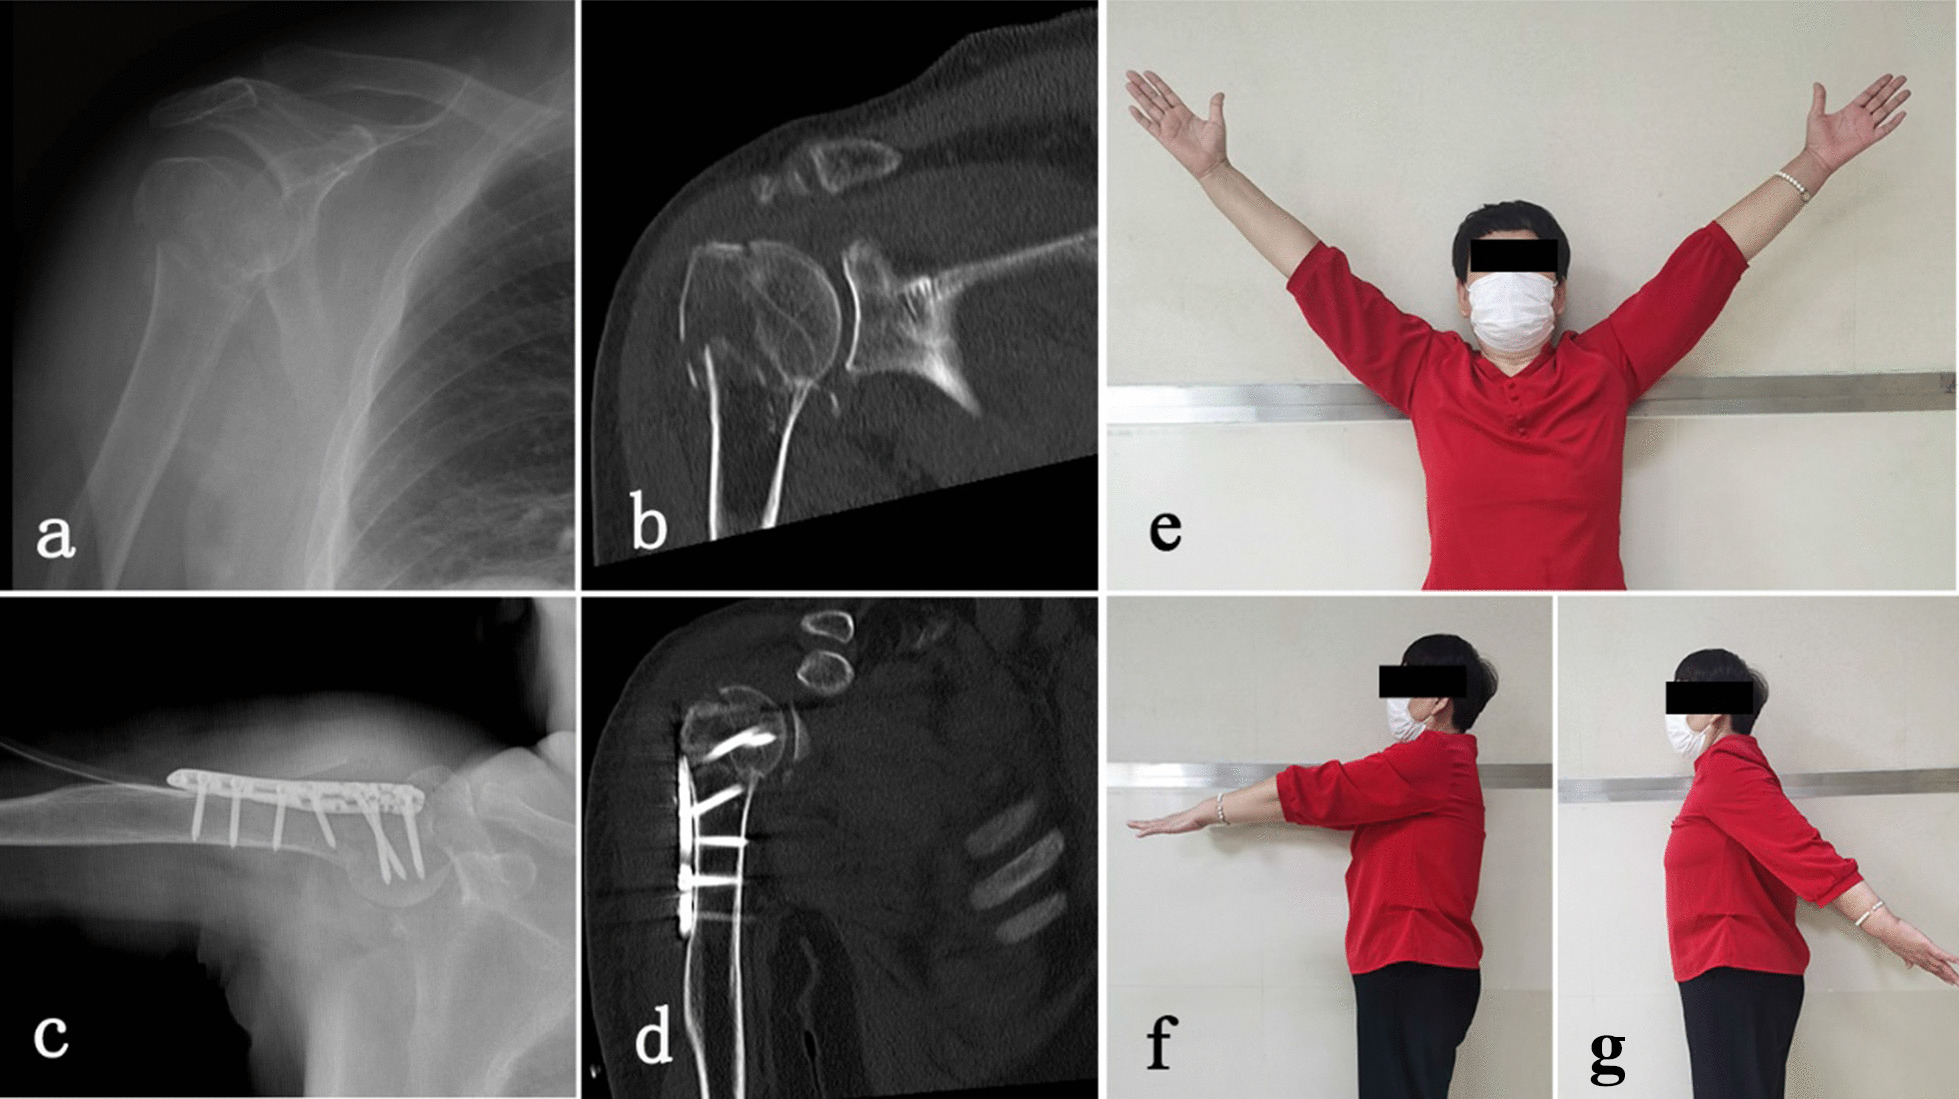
Patients with PHFs were treated using the locking plate approach. a Preoperative X-ray of the shoulder joint; b preoperative CT; c Postoperative X-ray; d Postoperative CT scan of the shoulder joint; e, f, j functional results at the final follow-up
